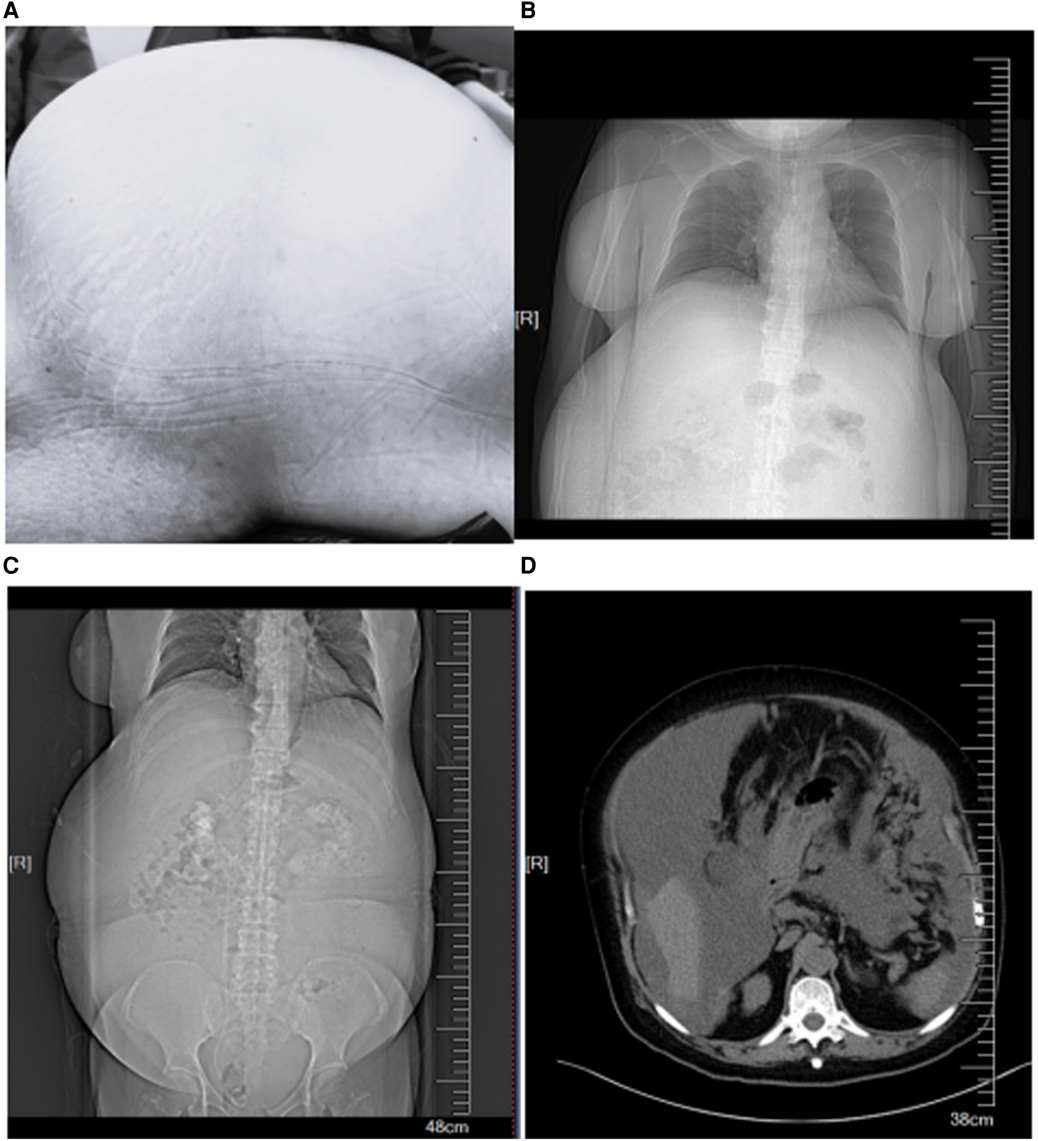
(A) The increased abdominal circumference. (B) Chest computed tomography revealed an elevated diaphragm. (C,D) Abdominal computed tomography revealed multiple cystic solid mass and nodular soft tissue density.
